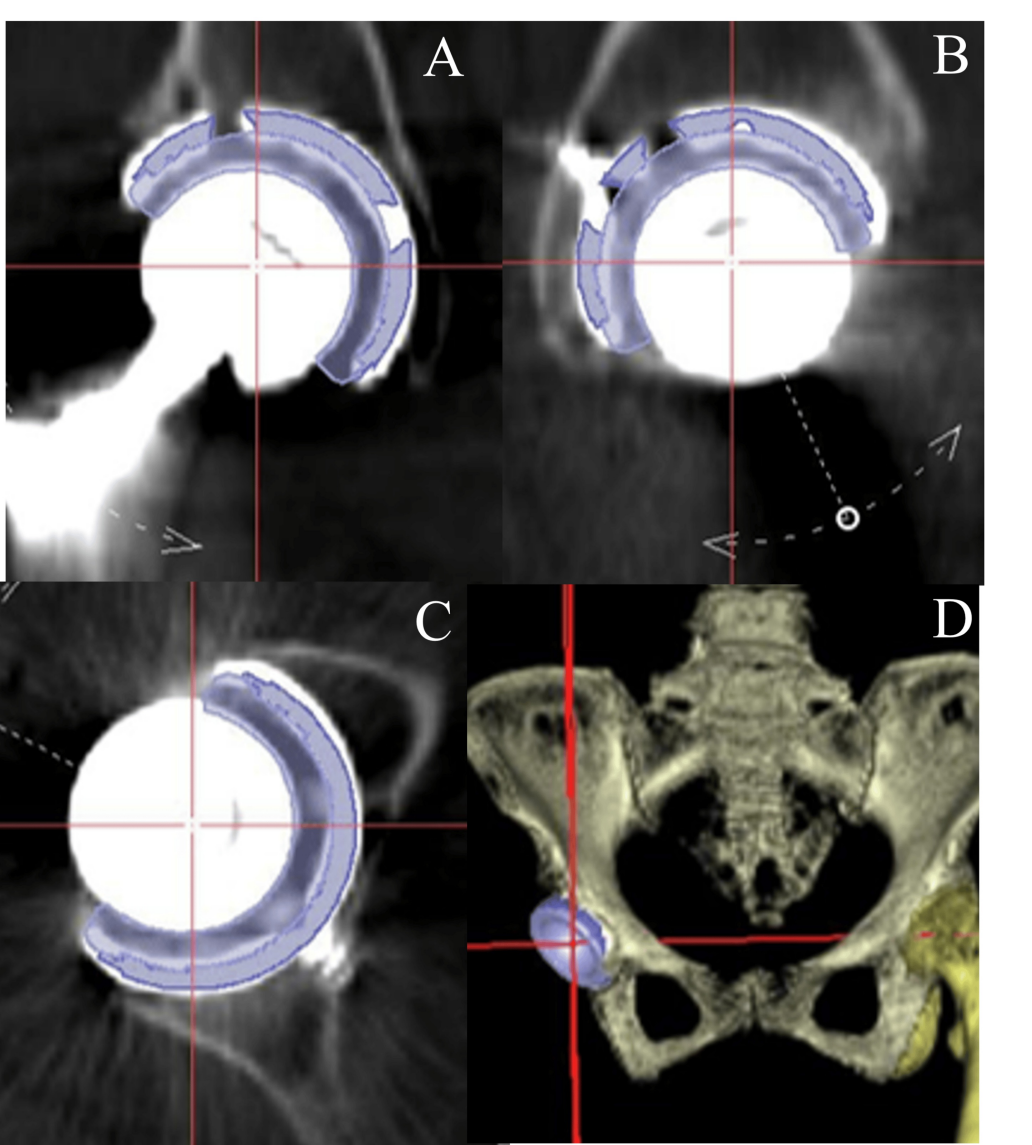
Extraction of coordinates for three-dimensional positioning of cups after surgery in same case with Figure 1 A: Longitudinal slice view B: Sagittal slice view C: Transverse slice view D: Three-dimensional CT
